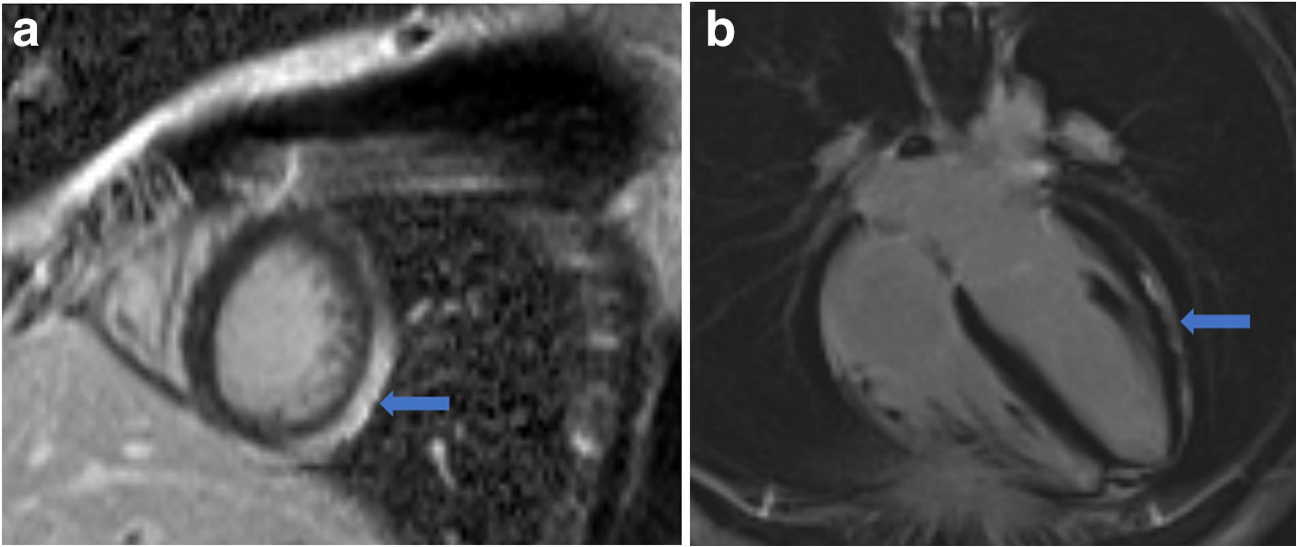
Myopericarditis in a patient with chest pain, ST elevation, and elevated cardiac troponins: a Two chamber view and b four chamber view post-contrast cardiac MRI demonstrate curvilinear delayed enhancement in the subepicardial lateral mid and apical wall of the left ventricle and the adjacent pericardium (blue arrows).
